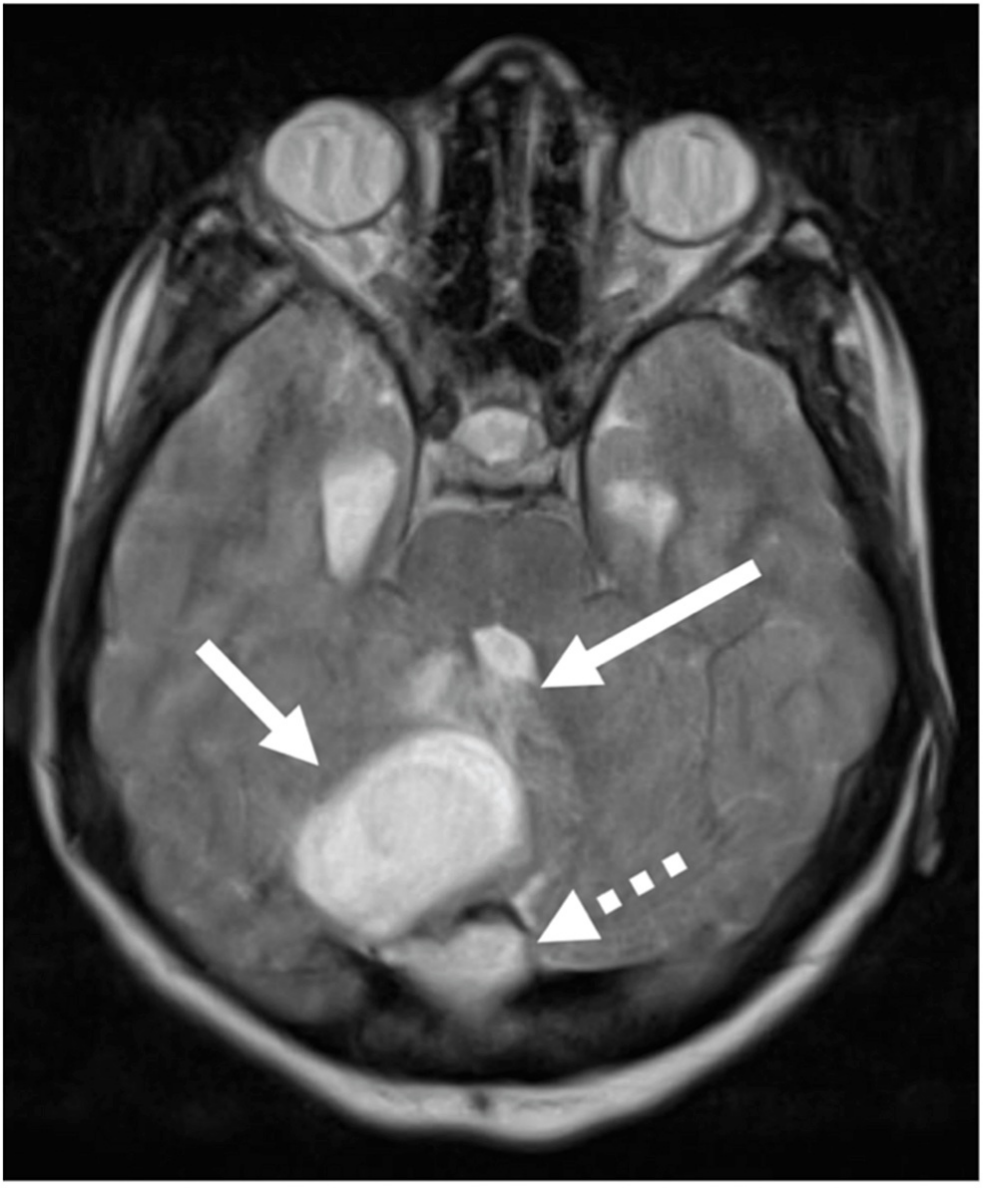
Axial T2WI showing the heterogeneous high-signal intensity of the cyst (short arrow) in the right cerebellar hemisphere, with a bilobar cyst dorsal to the posterior border of the original cyst (dashed arrow); focal surrounding edema and subsequent mass effect in the form of focal effacement of the fourth ventricle (long arrow). T2WI, T2-weighted image
